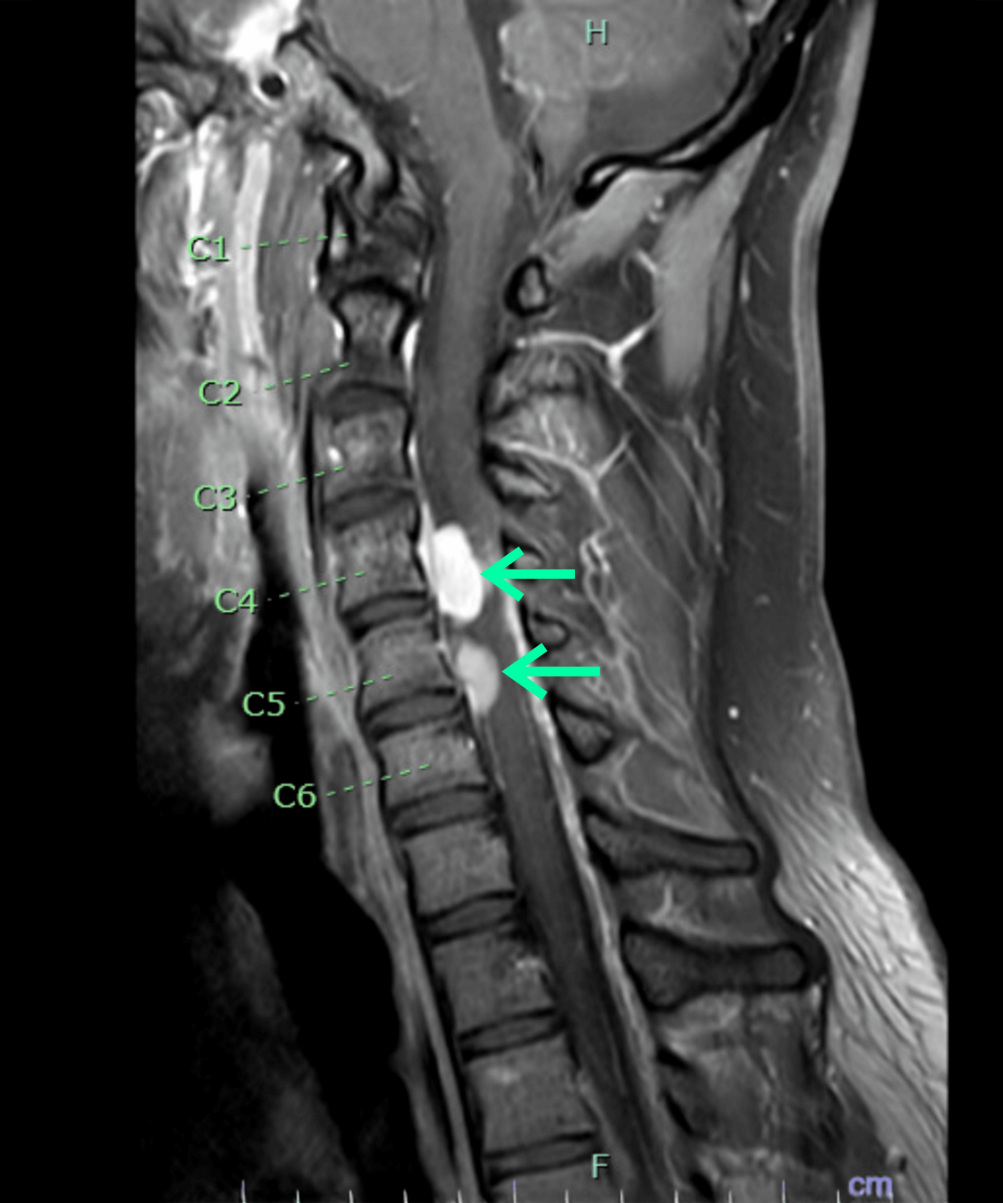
Cervical spine magnetic resonance imaging (MRI) showing two areas of tumor infiltration (myeloid sarcoma) involving the intradural extramedullary spaces (green arrows), measuring 18 mm and 13 mm at their largest dimensions.
